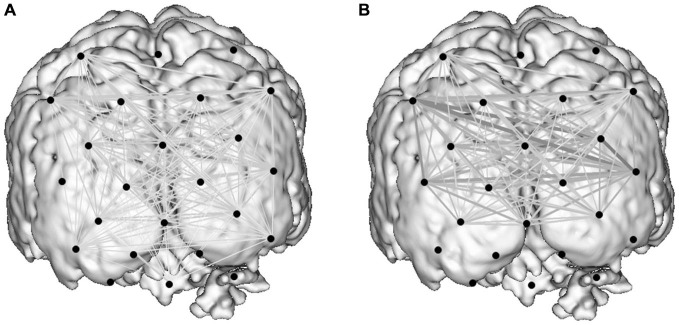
Activity-guided cortical networks for a representative subject during the control condition (A) and study condition (B). Approximate channel locations (black circles) are overlain onto reference MRI atlas. The strength of functional associations between nodes in the network is represented by the boldness of network edges.
